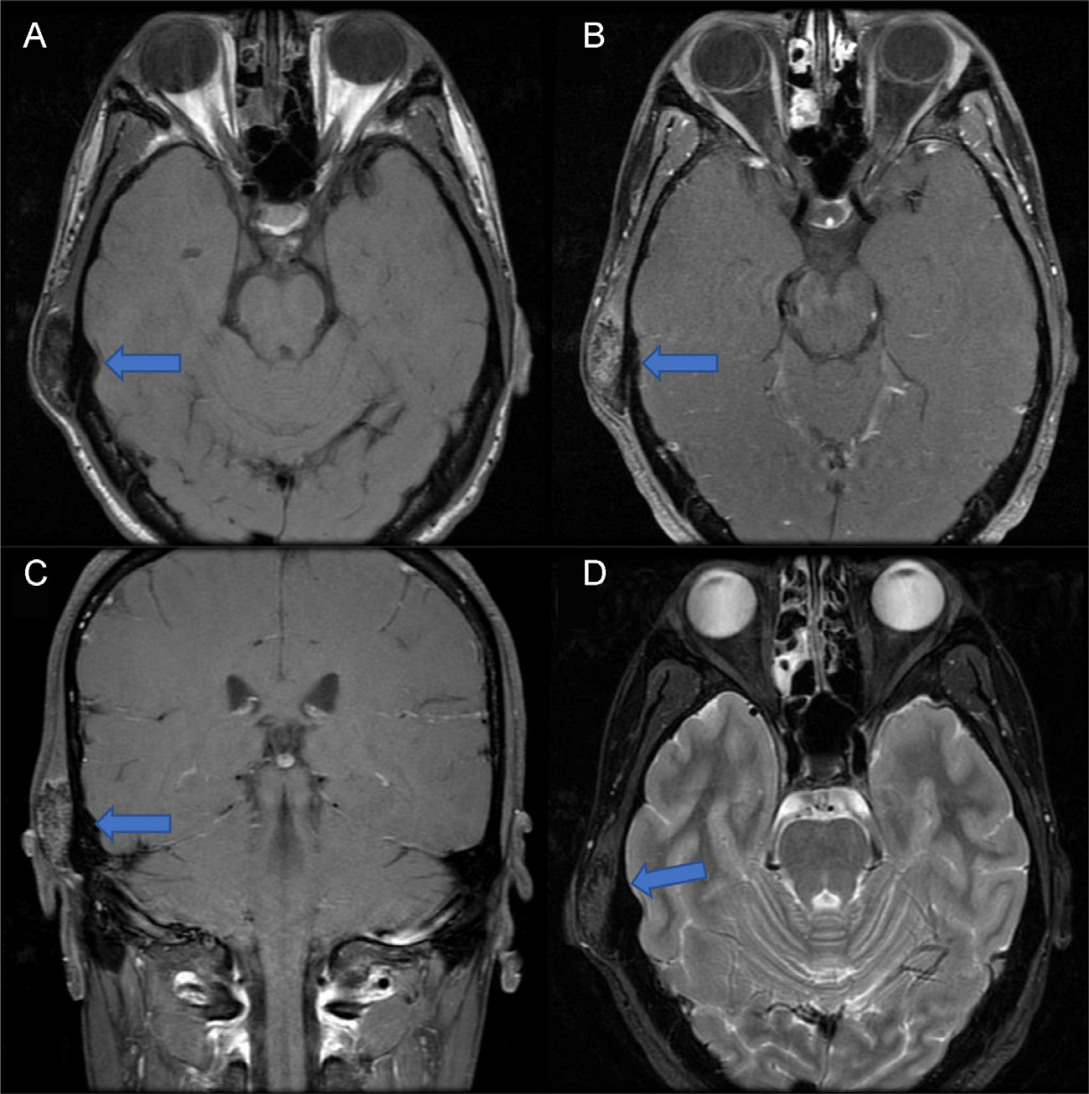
(A-D) MRI with (A, C) and without (B) contrast showed a densely calcified mass measuring 3.6 × 1.5 × 3.0 cm (blue arrows) that involves the inferior aspect of the squamosal portion of the right temporal bone and is located directly superior to the petrous portion. The lesion has a low T1 (A-C) and T2 (D) signal intensity which originates from the outer table and extends into the soft tissues with mild patchy internal enhancement. Thickening of the inner table of the calvarium is present in the same region with no abnormal signal intensity or enhancement. There is no soft tissue component present beyond the bone. No dural involvement or any other intracranial structures. A small amount of fluid in the right mastoid air cells is present. Mucosal thickening present in the right ethmoid air cells.
